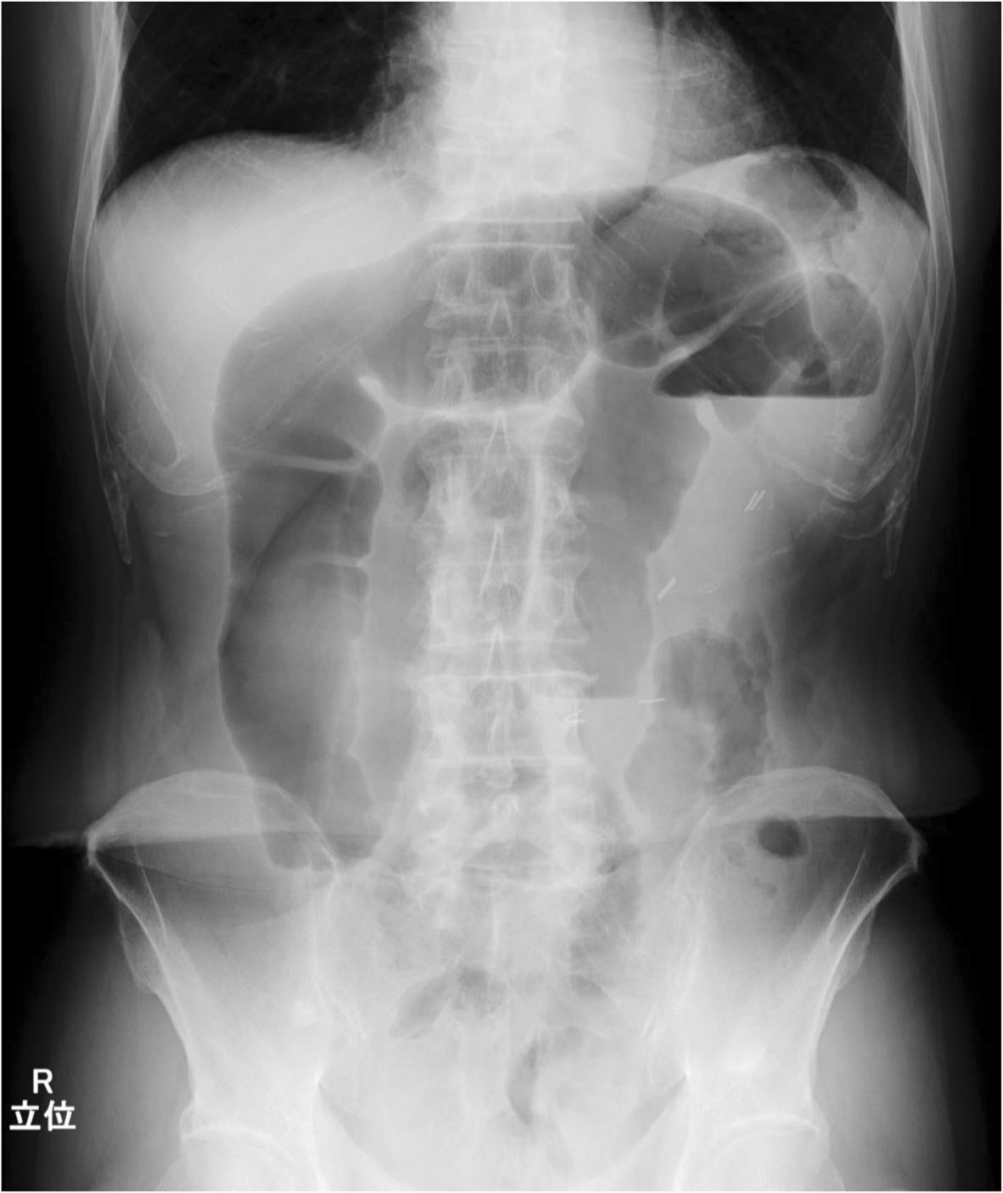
Intestinal gas was predominant on an abdominal X-ray
